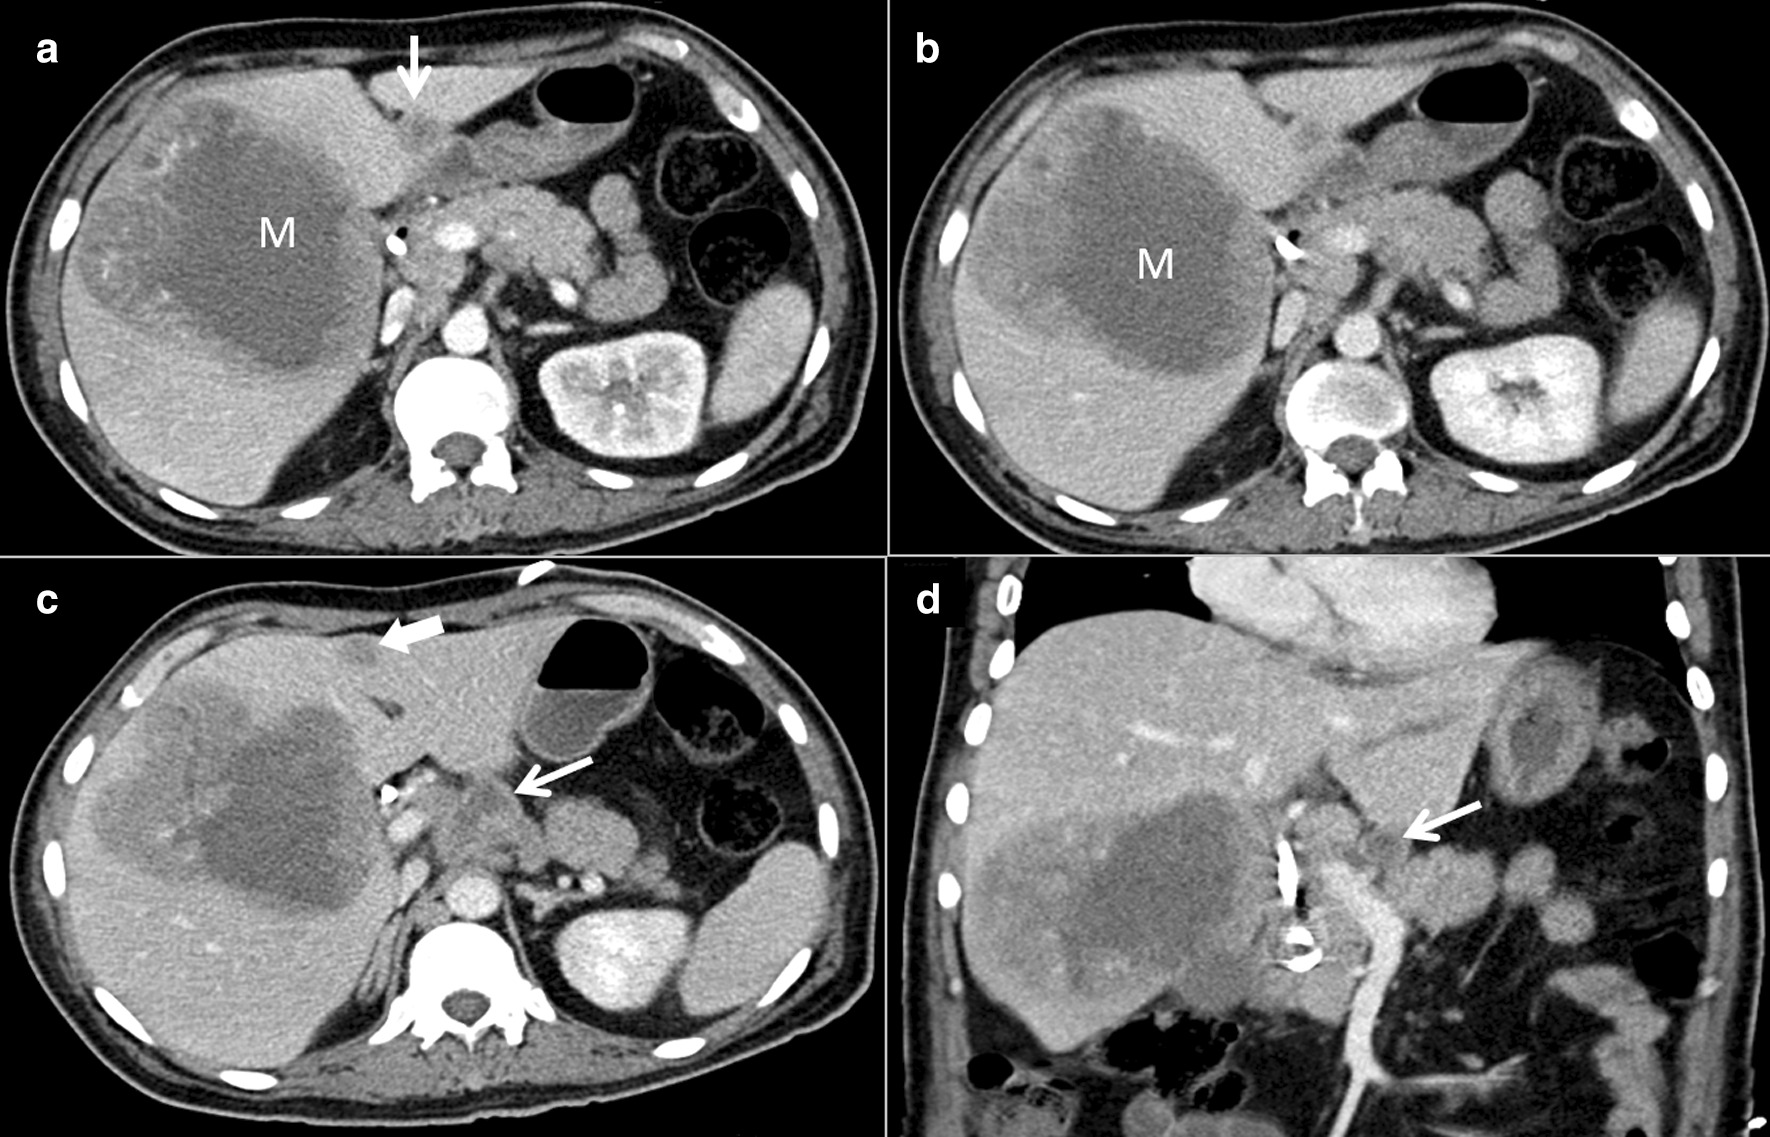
Contrast-enhanced abdominal computed tomography images acquired 1 month following the initial presentation (a axial image in the arterial phase; b, c axial images in the venous phase; and d coronal image in the venous phase) demonstrating an interval increase in the size of the mass lesion (M) with larger central hypodensities (a and b). Necrotic lymph nodes (thin arrows in c and d) were observed. A new nodule in the left liver lobe (thick arrow in c) and interval increase in the size of the nodule at the falciform ligament (arrow in a) were noted
